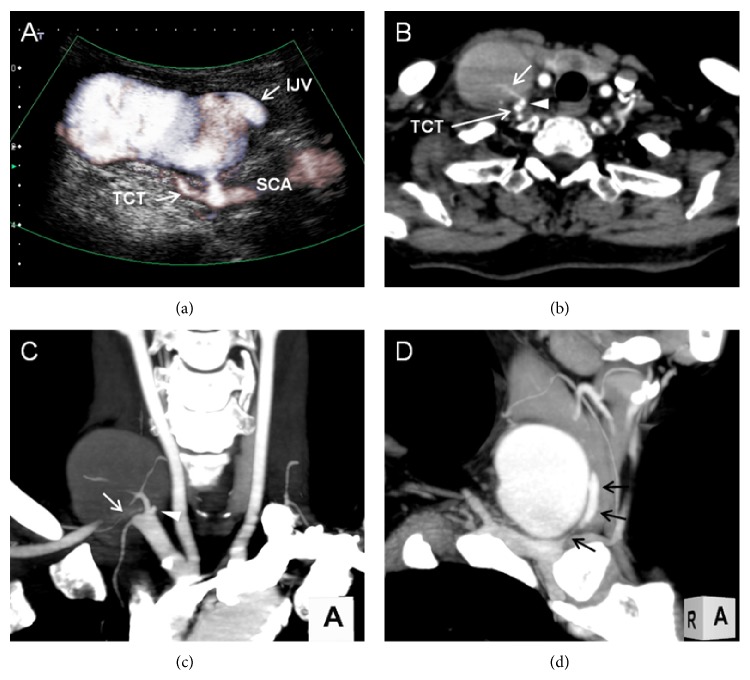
(a) Duplex ultrasound in the longitudinal plane at the level of the right supraclavicular fossa. The PsA communicated with the root of the thyrocervical trunk (TCT) with inner turbulent flow via the short, narrow neck. Continuity between the PsA and the internal jugular vein (IJV) was also noted. SCA: subclavian artery. (b) Contrast-enhanced computed tomography angiography showed a high-density-flow jet of contrast agent shunting from the TCT (arrow) into the aneurysmal sac via the short neck (arrowhead). (c) Maximum-intensity projection (MIP) image in the arterial phase clearly showed the anatomical relationship between the TCT and PsA neck (arrowhead). A flow jet within the PsA was also shown (arrow). (d) MIP image in the venous phase showed continuity between the aneurysmal sac and internal jugular vein (arrows).
